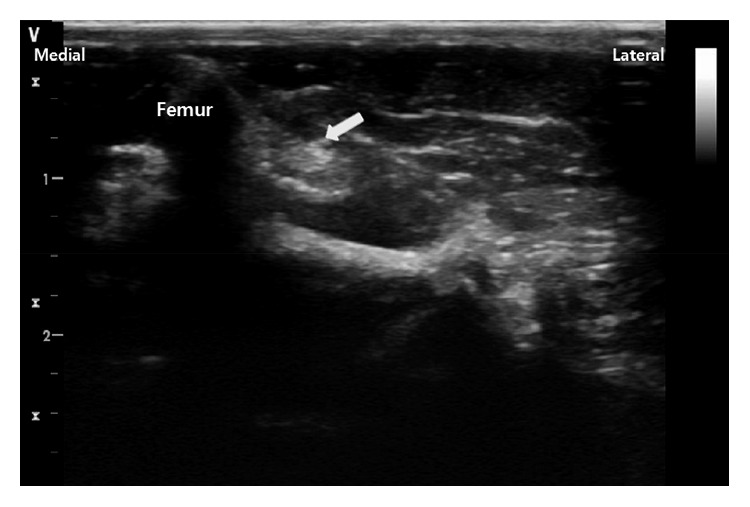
Ultrasound image of sciatic nerve (arrow).
